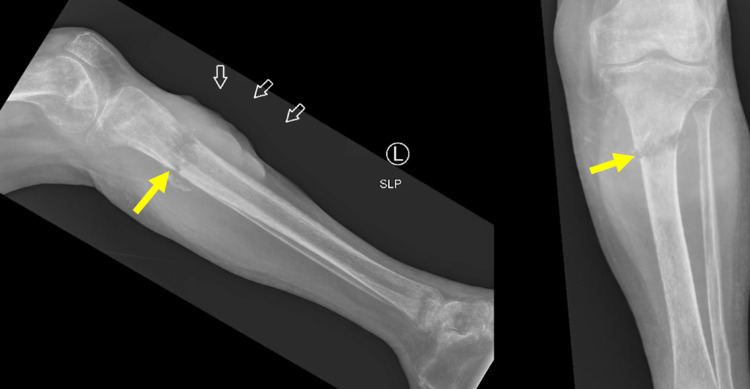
Radiographs from the time of diagnosis of proximal tibial lymphoma, five months after the patient suffered an atraumatic leg injury. The fracture (yellow arrows) appears as a transverse band of lucency with ill-defined margins. A soft tissue mass (white arrows) is also apparent.
